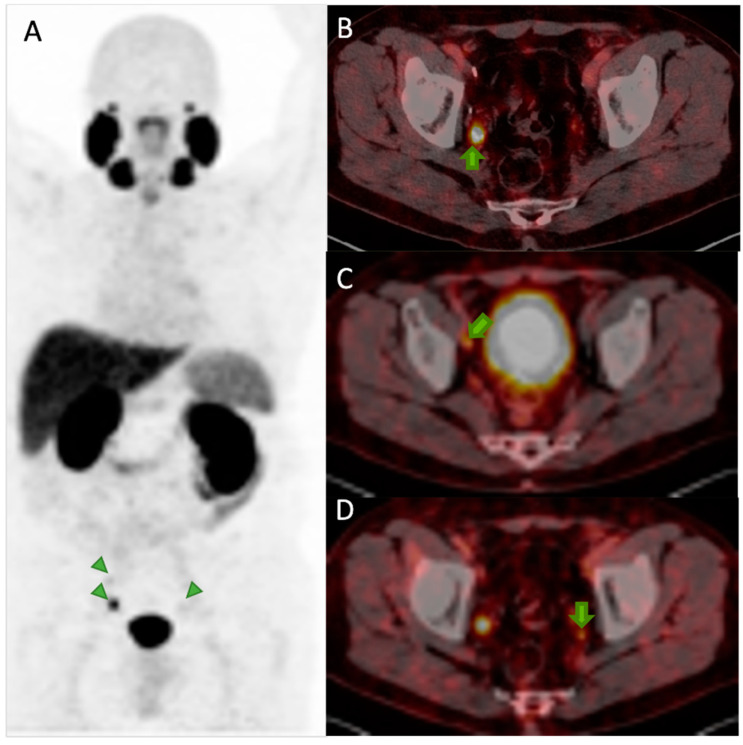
PSMA PET/CT of a patient undergoing restaging due to biochemical recurrence of prostate adenocarcinoma (Gleason 3 + 4; PSA = 0.8 ng/mL). (A) The MIP image shows multiple bilateral pelvic lymph node metastases with hyperexpression of PSMA receptors (arrowheads). The fused axial images demonstrate the metastases (green arrows) located in (B) a right internal iliac lymph node (0.6 cm; SUV = 11), (C) a right external iliac lymph node (0.5 cm; SUV = 3.4) and (D) a left internal iliac lymph node (0.3 cm; SUV = 4.2). There were no signs of local recurrence.
